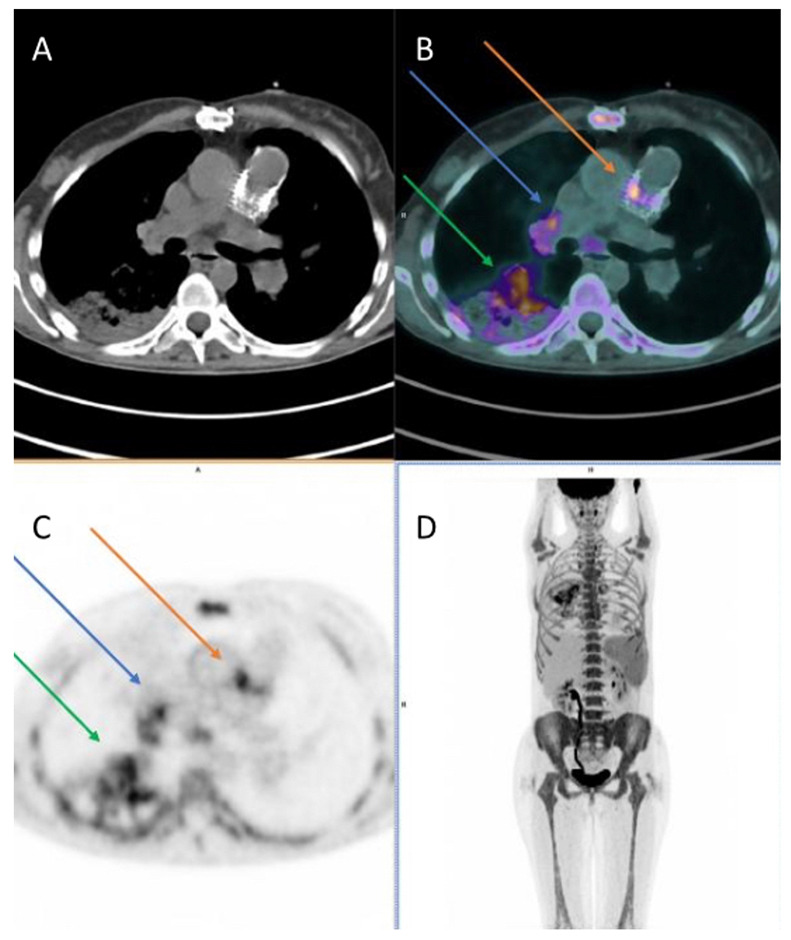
A case of pulmonary valve/valve duct endocarditis of a 38-year-old female patient (surgically treated for Fallot tetralogy in 1988 and 2012), evaluated with ^18^F-fluorodeoxyglucose (^18^F-FDG) positron emission tomography/computed tomography (PET/CT): axial CT, PET/CT, and PET images (A–C) and maximum intensity projection (MIP) image (D) show hypermetabolism in the pulmonary valve prosthesis and in the valve duct (orange arrows), with extension to aortic wall (demonstrating a disease involvement in the aorta as well). Moreover, the images depict multiple reactive hylo-mediastinal lymph-nodes with high uptake of the radiopharmaceutical (blue arrows) and hypermetabolism in an area of consolidation in the right lung (manifestation of pneumonia, green arrows). In addition, image D shows high uptake of ^18^F-FDG in spleen and bone marrow, as a sign of systemic inflammatory response.
